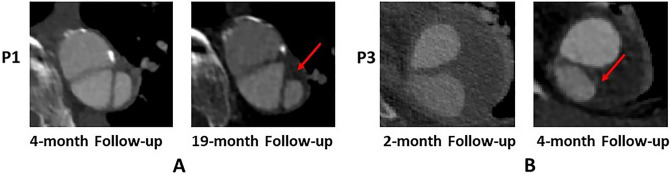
Partial thrombosis observed on CT scans in P1 (A) and P3 (B) at the subsequent follow-ups. Locations of thrombus formation are indicated by red arrows.
